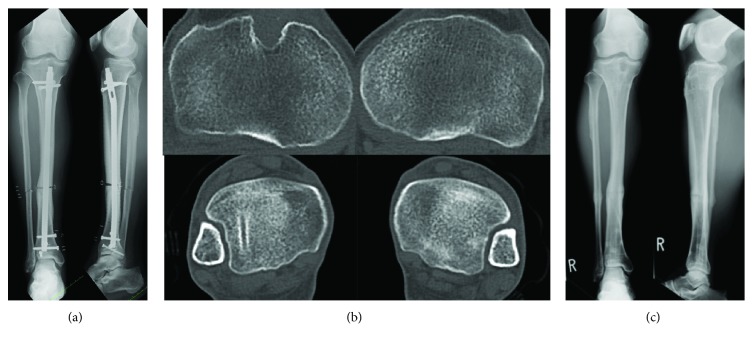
(a) Anteroposterior and lateral radiographs of the right tibia obtained immediately after corrective osteotomy. (b) CT images of the right and left tibias obtained 1 year after corrective osteotomy. (c) Anteroposterior and lateral radiographs of the right tibia obtained 5 years after corrective osteotomy.
